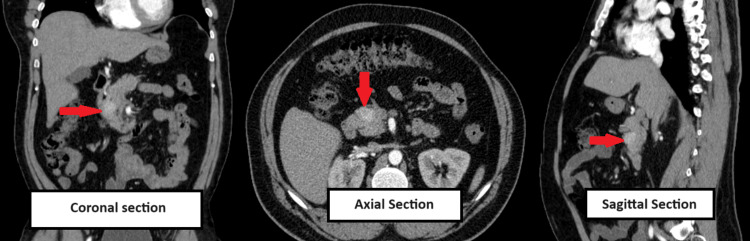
CECT showing small ill-defined lesion with mild arterial enhancement in the head of pancreas raising suspicion of neuroendocrine tumour of pancreas. Red arrow indicates lesion in pancreas. CECT: contrast-enhanced computed tomography
